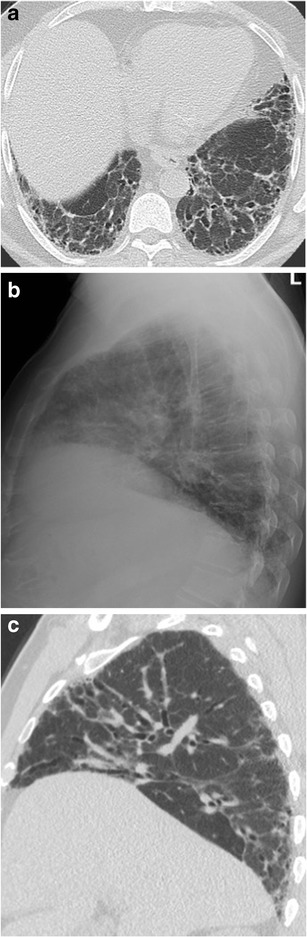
68-year-old man with idiopathic pulmonary fibrosis. An axial CT slice (a) shows fibrosis at the lung bases. On the lateral radiograph (b), reticular opacities are seen in the retrosternal clear space but not at the lung bases, where they are obscured by pulmonary vasculature and the spine. A corresponding sagittal CT slice (c) is also shown
